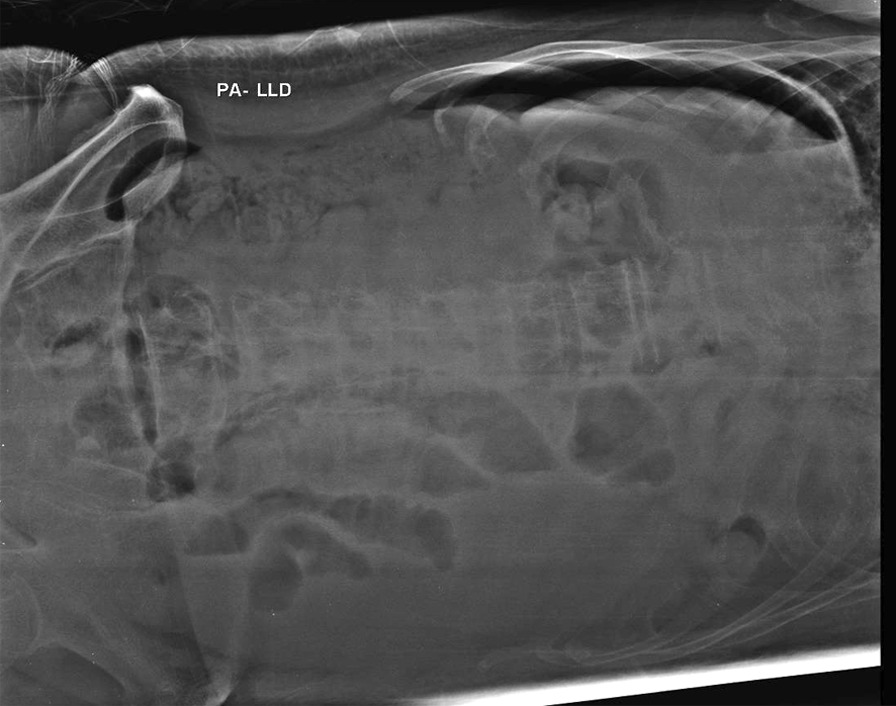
Abdomen X-ray in left lateral decubitus showing free air in the abdominal cavity
